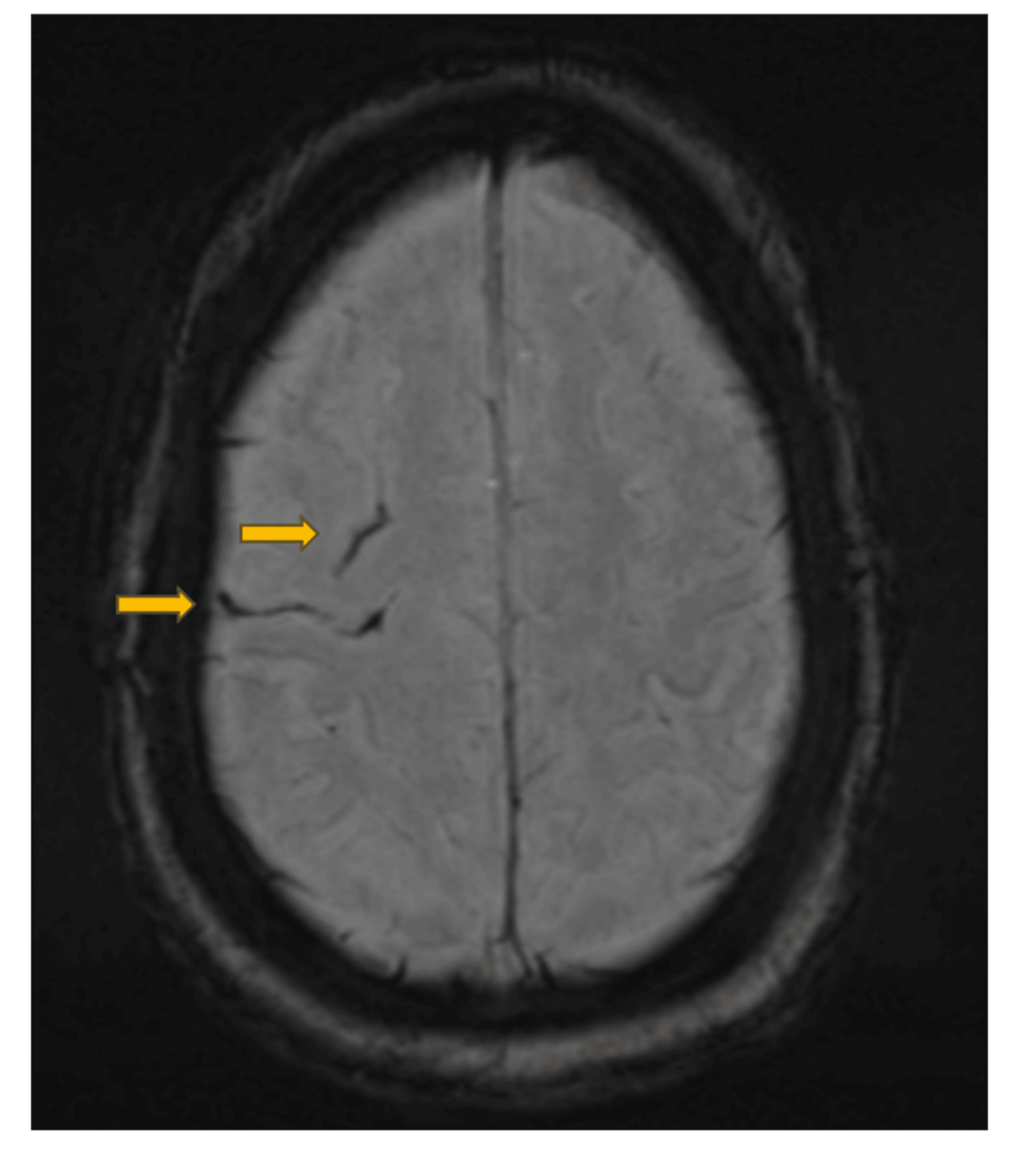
Magnetic resonance imaging (MRI) of the cerebrum Arrows show subarachnoid hemorrhages in two sulci in the right frontal lobe
